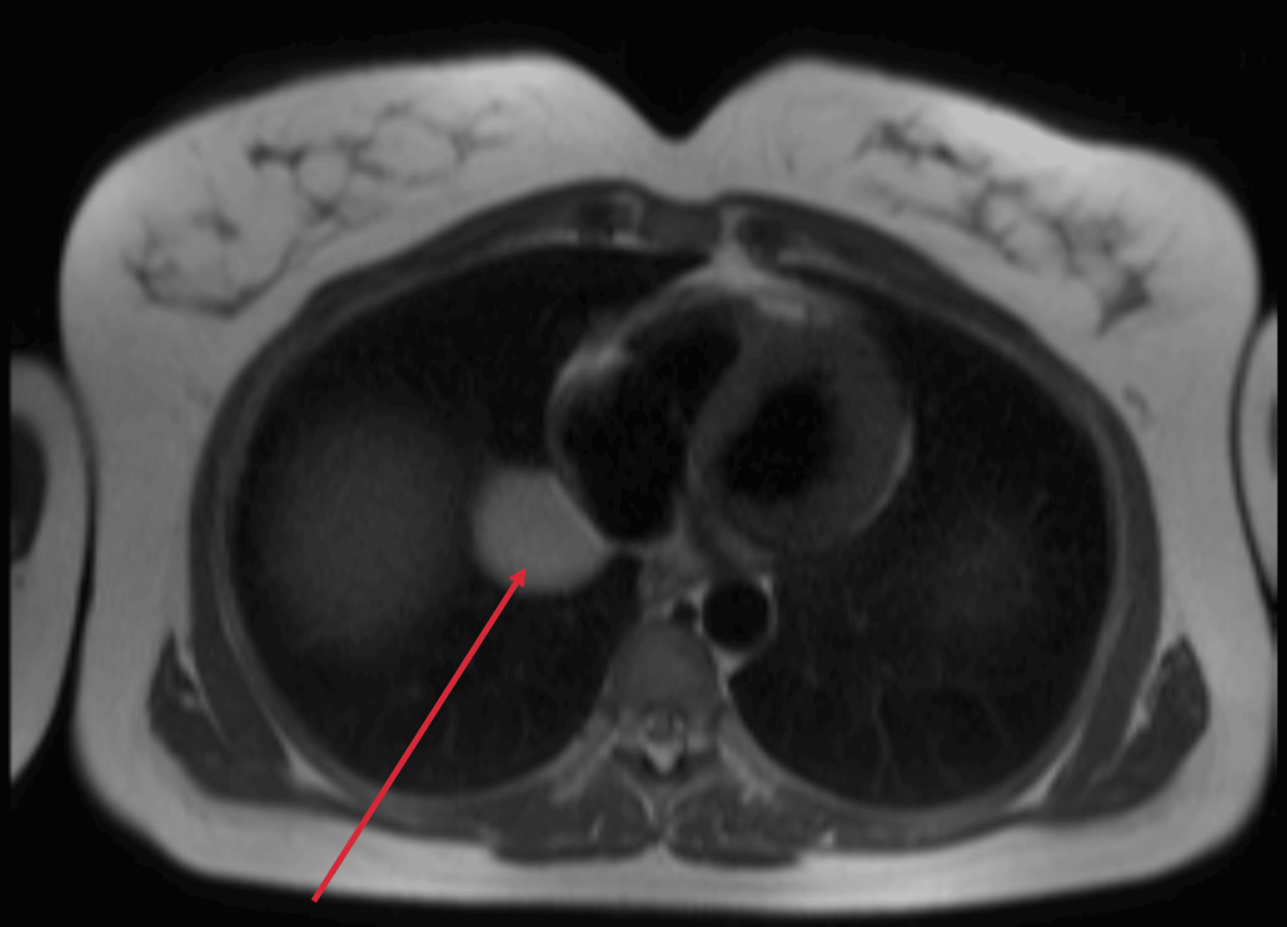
Transverse view of the cardiac MRI. The red arrow points to the pericardial cyst.
